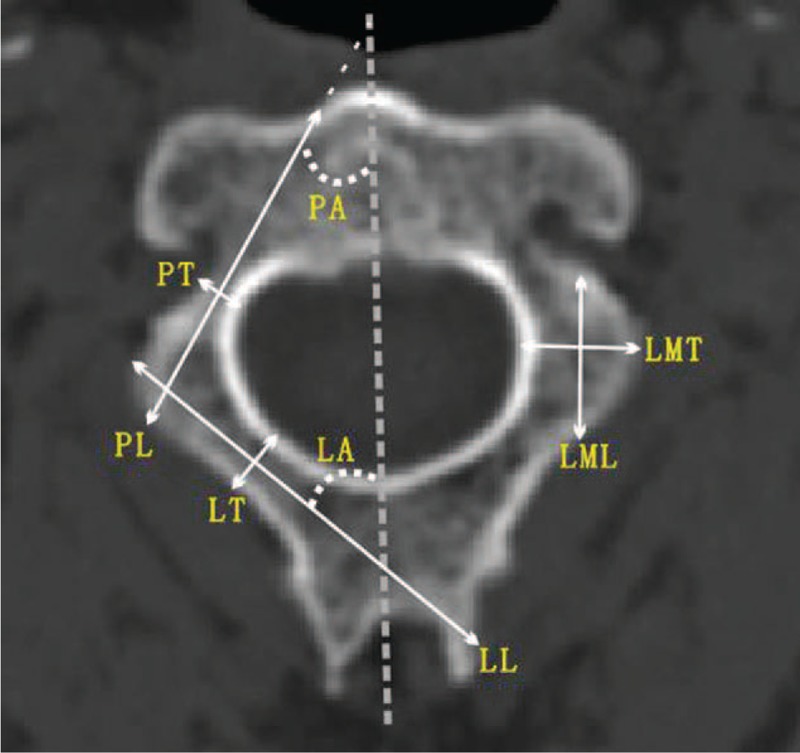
Measurements of C2 on the axial CT image. CT = computer tomography, LA = laminar angle, LL = laminar length, LML = lateral mass length, LMT = lateral mass thickness, LT = laminar thickness, PA = pedicle angle, PL = pedicle length, PT = pedicle thickness.
